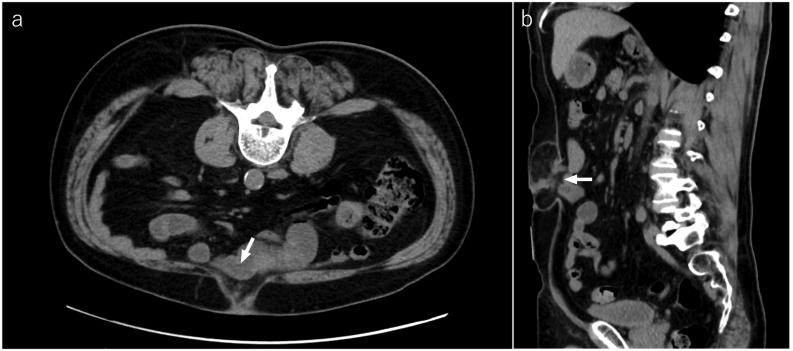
Abdominal computed tomography in the prone position before enhanced-view totally extraperitoneal repair demonstrates an incisional hernial orifice in the umbilicus. a) Axial image showing a hernia defect measuring 5 cm in width (arrow). b) Sagittal image showing a hernia defect measuring 4 cm in length (arrow).
